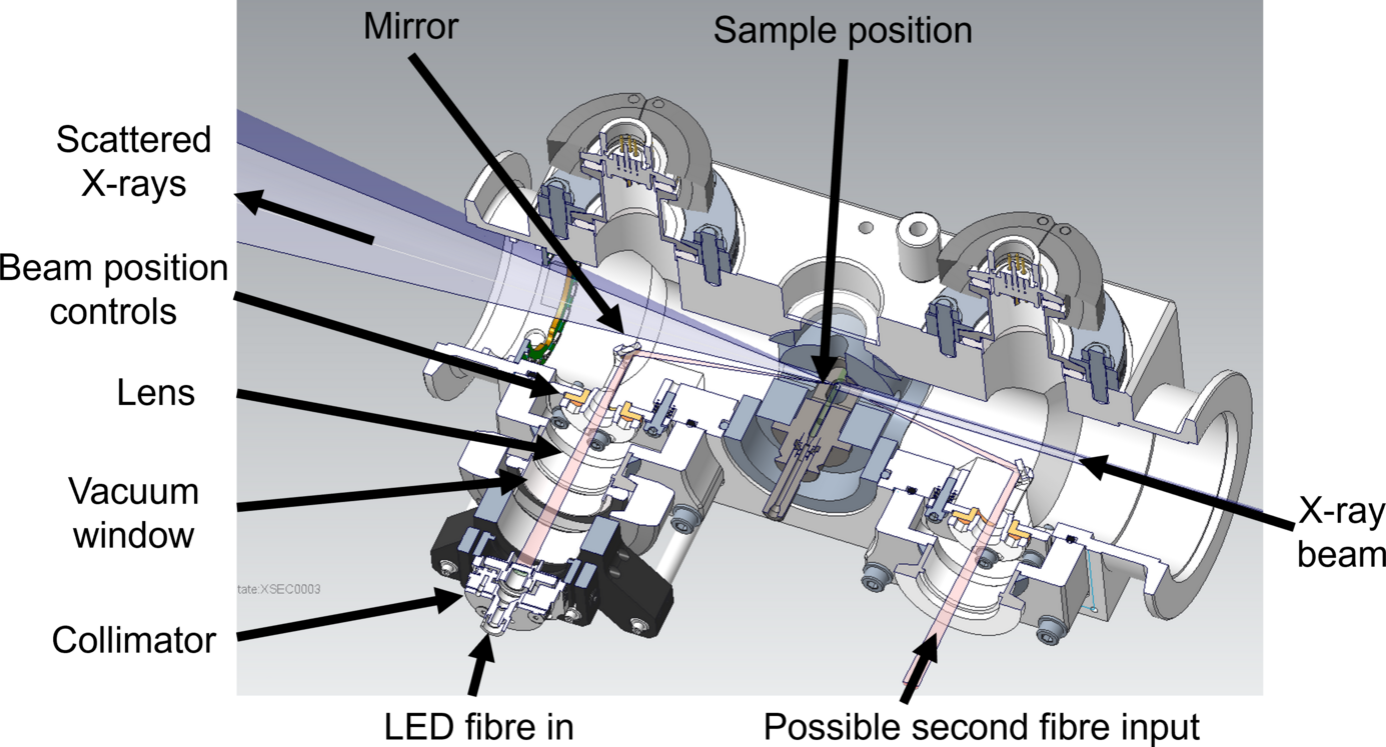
Design for the *in situ* irradiation environment. The X-ray beam is incident from the right. The LED fibre input is downstream from the sample position; the light beam travels through a series of focusing optics before being reflected, parallel to the X-ray beam, onto the sample. The design has flexibility for a second fibre input upstream from the sample position, but this is not currently in use.
